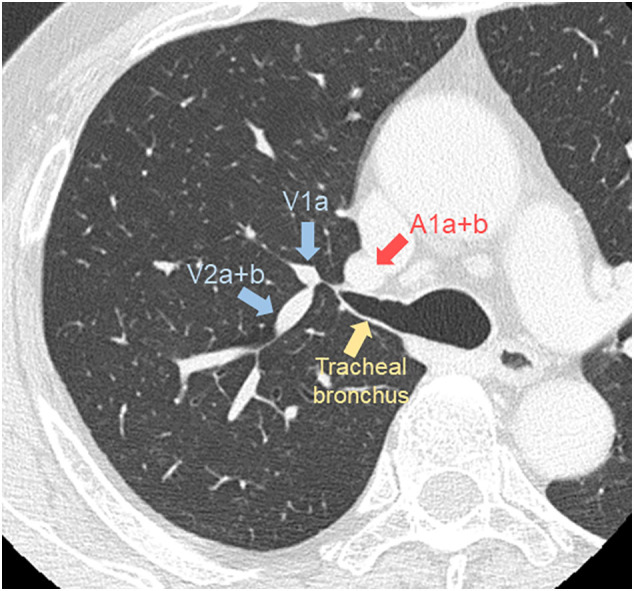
Axial CT slice at the level of the tracheal bronchus demonstrating the locations of A1a + b, V1a, and V2a + b.
